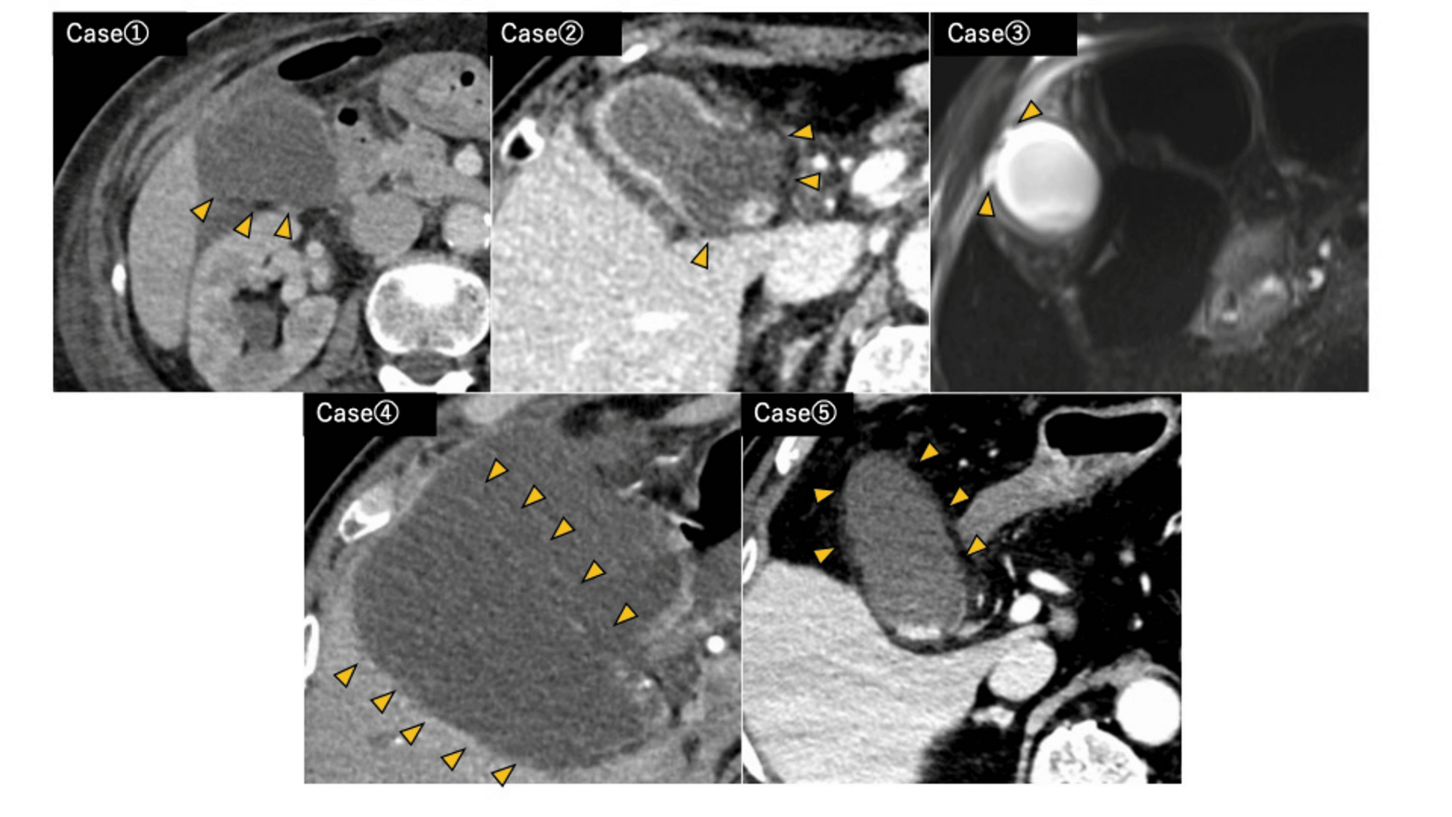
Preoperative CT or MRI findings Cases 1, 2, 4, and 5: Contrast-enhanced CT showing reduced enhancement of the gallbladder wall at the areas indicated by the arrowheads. Case 3: MRI T2-weighted image showing a high-intensity area seen from the gallbladder fundus along the abdominal wall, suggesting bile leakage
